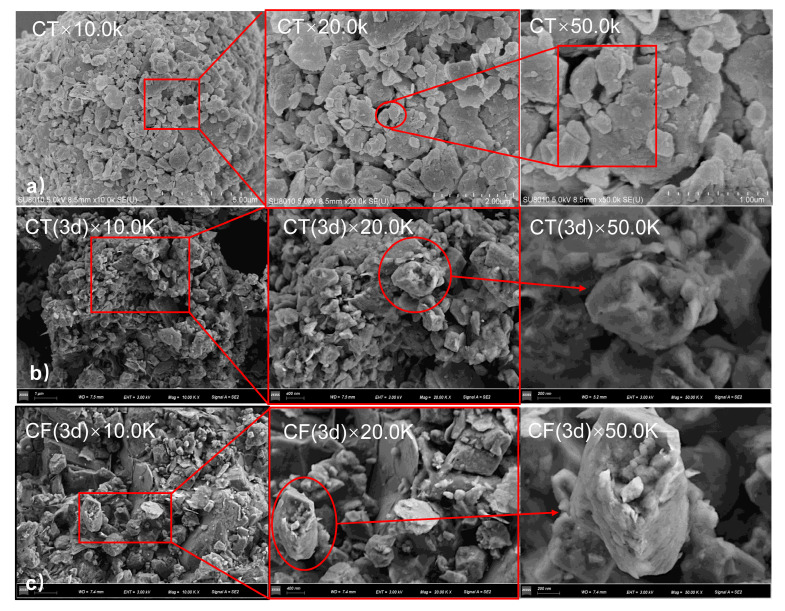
(a): SEM images of CT before sorption; (b): SEM images of CT with a sorption time of 3 d; (c): SEM images of CF with a sorption time of 3 d.
